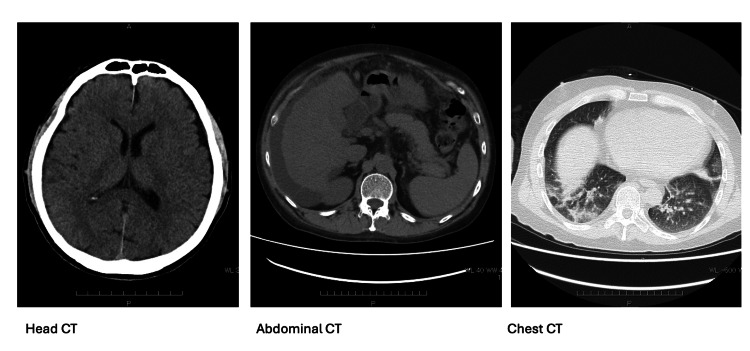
CT scan of the head, abdominal, and chest (axial views) on admission. Head CT (axial view) shows no acute intracranial changes, and abdominal CT (axial view) demonstrates hepatic cirrhosis with mild ascites, consistent with underlying chronic alcohol use. Chest CT (axial view) demonstrates pneumonia.
